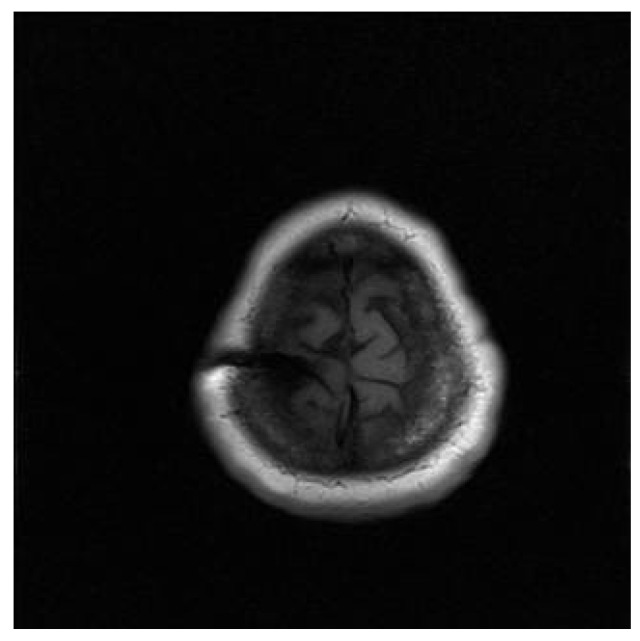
Preoperative T1 MRI sequence showing a previous brain convexity placement of an electrode at the coronal region.
